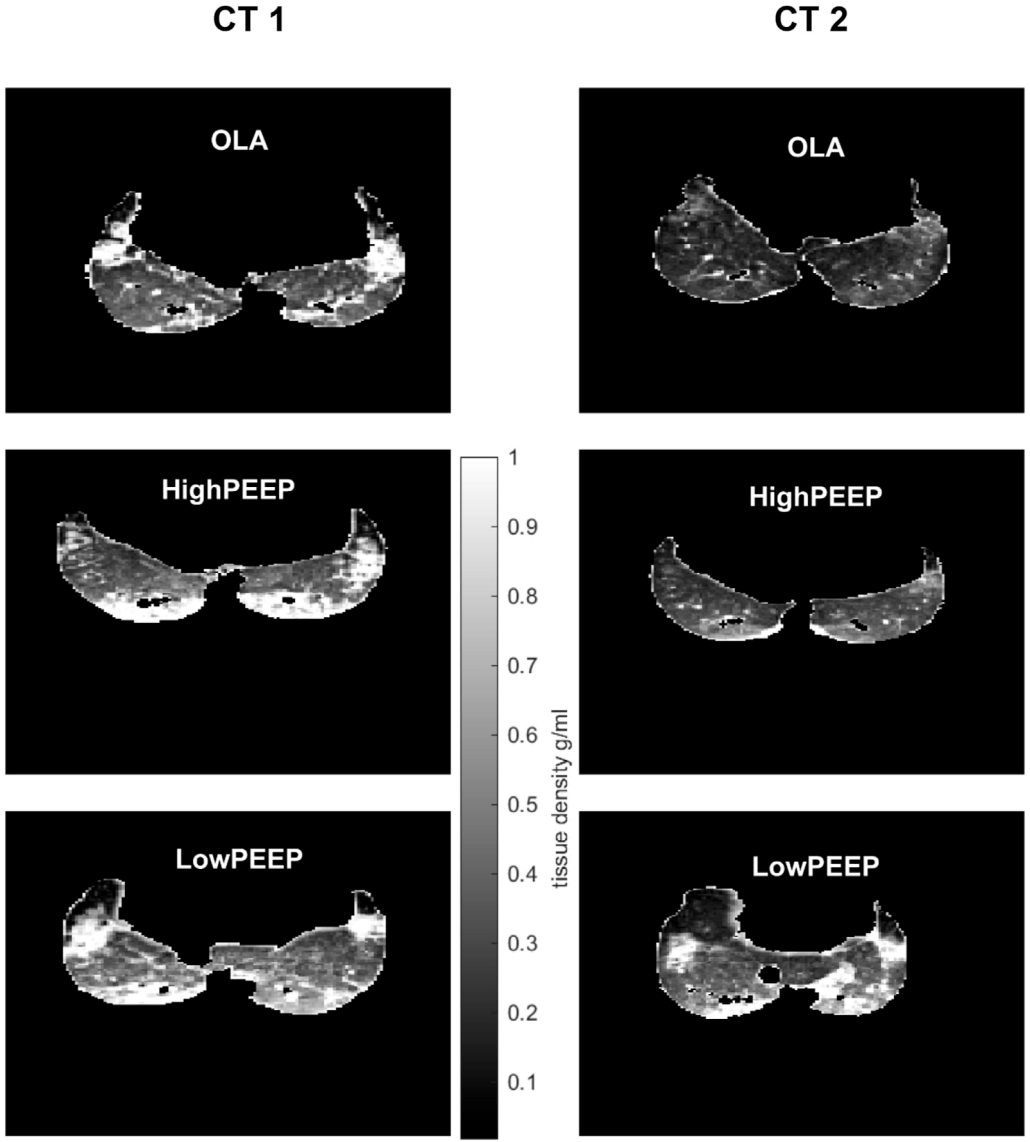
Representative single-slice images of lung density with ACCT. According to the density color bar, hyper-density with lower aerated compartments, i.e., atelectasis, are indicated by white color, while hyper-aerated compartments, i.e., airways, are indicated by black color. CT 1, at the start of intervention; CT 2, at the end of intervention.
